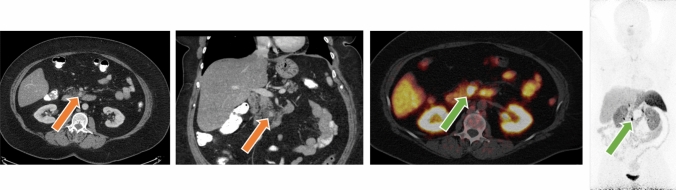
Computed tomography (CT) of the abdomen with intravenous (IV) contrast of a ~ 1 cm non-functioning PNET in the uncinate process (*orange arrows*) under active surveillance and without evidence of locoregional or distant metastases on DOTATATE PET CT (*green arrows*). PNET, pancreatic neuroendocrine tumor; PET, positron emission tomography
